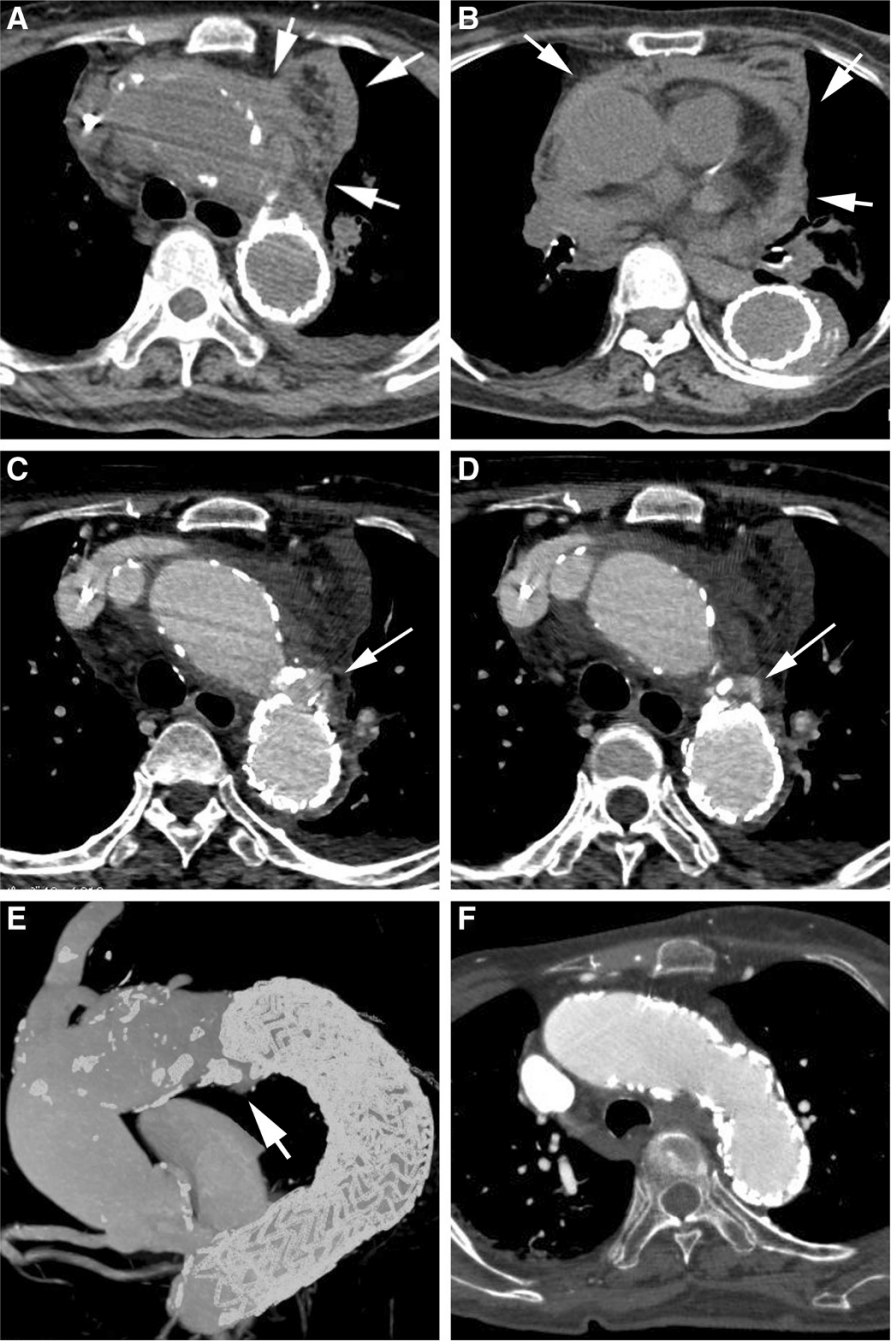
An 87-year-old female with type B acute intramural hematoma of the aorta (IMH). One month later form onset, TEVAR (GORE TAG: 37-mm diameter & 20-cm length) was performed because of ULP enlargement. A, B. One year after TEVAR, this patient complained of severe chest pain. Unenhanced CT images show a mediastinal hematoma (arrow). C-E. Contrast-enhanced CT and MIP images show that a SINE tear (small pseudoaneurysm: arrows) due to the edge of the stent-graft was noted. Re-TEVAR (GORE TAG: 37-mm diameter & 20-cm length × 2) was performed to close a SINE tear. F. Three months after re-TEVAR, a SINE tear and mediastinal hematoma disappeared.
